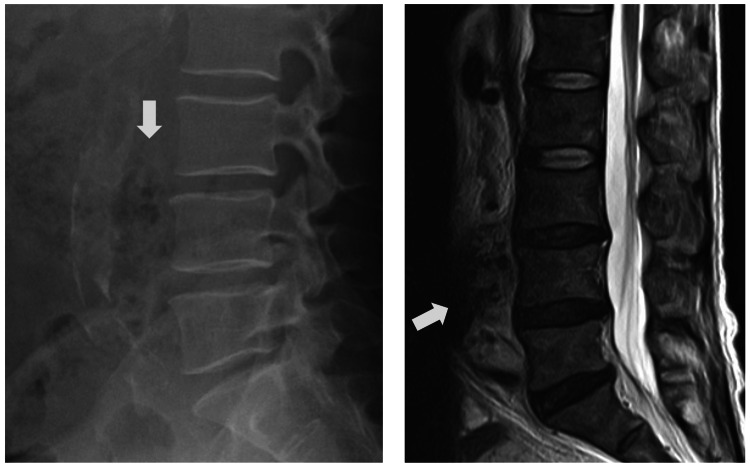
Radiographic and magnetic resonance imaging (MRI) of the lumbar spine before treatment. (A) Lumbar spine radiograph and (B) MRI showing no findings suggestive of discitis or vertebral body destructive lesions at the time of the initial examination. We observed the presence of an imminent rupture of the infected abdominal aortic aneurysm (white arrow) on reexamination.
